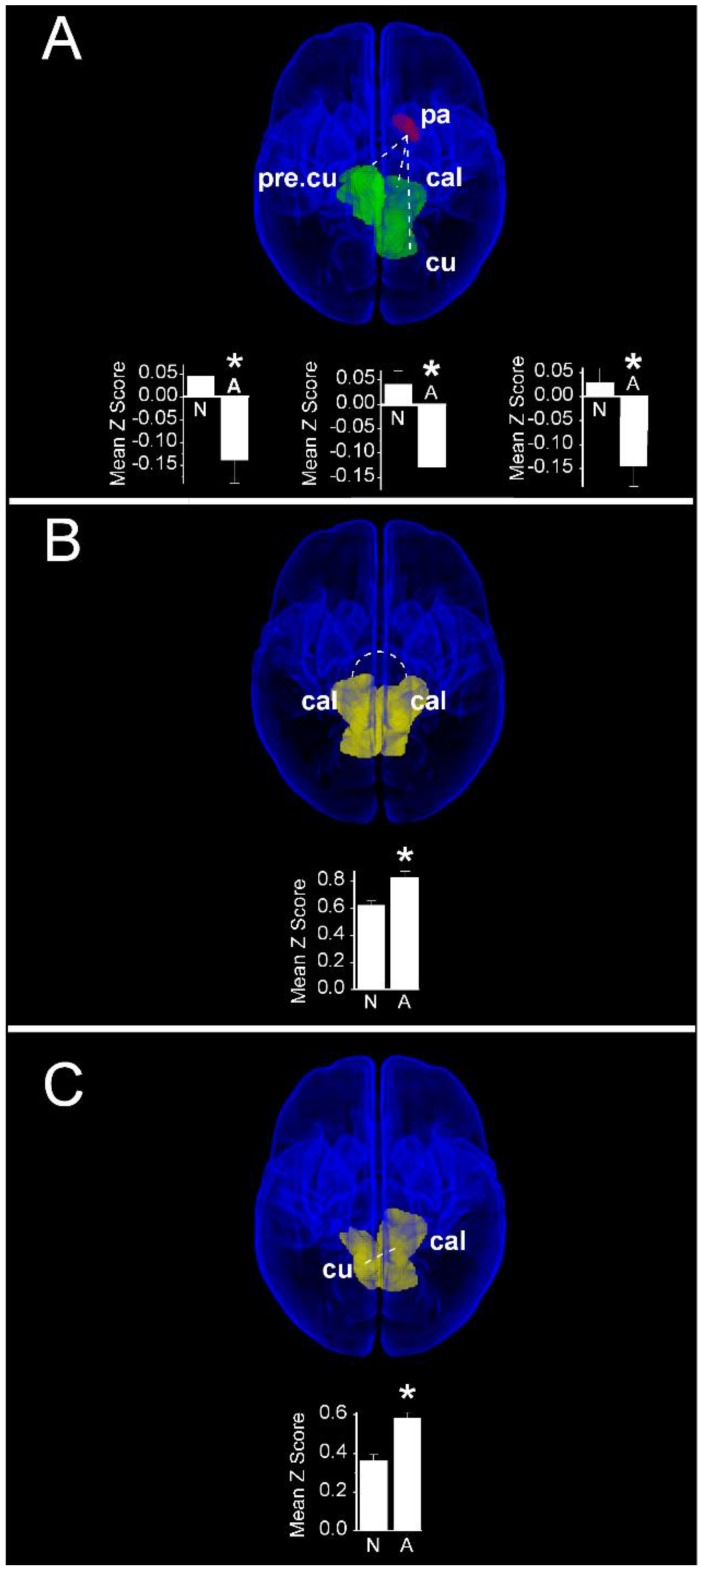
Region pairs exhibiting increased and decreased functional connectivities in infants with normal (≥85) and abnormal (<85) scores on the motor subscale of the Bayley III assessment at two years post-menstrual age. Obliquely projected 3D reconstructions of MRI images of cerebral grey matter and identified parcellated regions derived from a neonatal atlas. Dashed white lines depict connectivity relationships. Inset: Fisher-transformed Z-scores ± 1 SEM. (A) Increased connectivity between pallidum and visual cortex regions: left: left pallidum and contralateral precuneus; middle: Left pallidum and ipsilateral calcarine cortex; and right: Left pallidum and ipsilateral cuneus. (B) Decreased connectivity between bilateral calcarine cortices; (C) Decreased connectivity between left calcarine and right cuneus. N: Normal; A: Abnormal. * *P*_FDR_ < 0.05.
